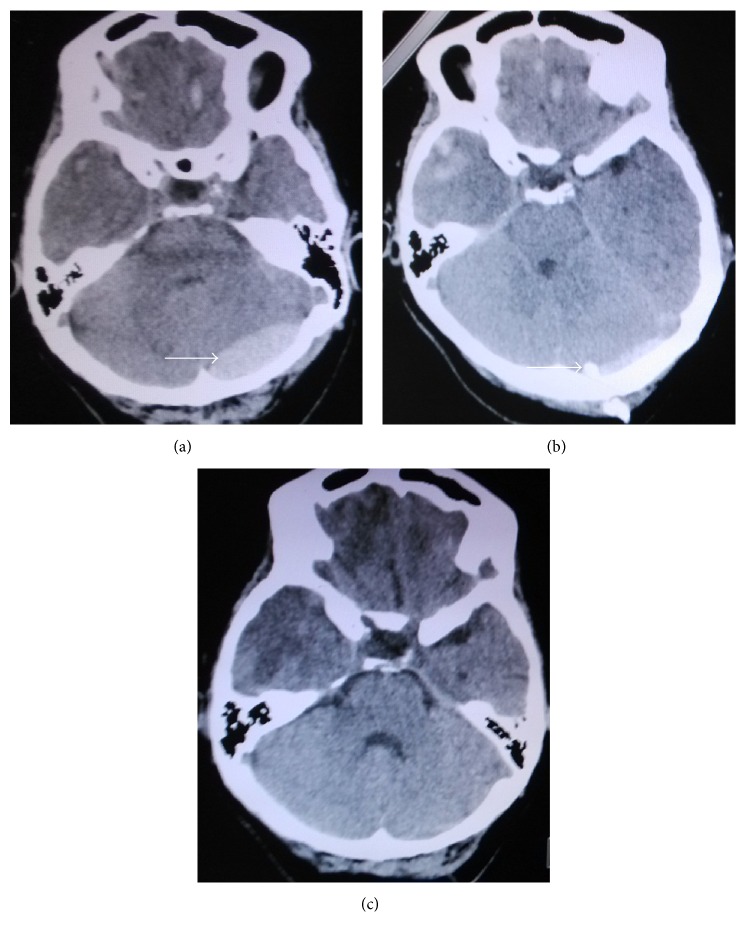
(a)–(c) Head CT images showing acute epidural hematoma at the left occipital region prior to and after surgery. (a) Head CT images prior to surgery; bleeding volume was approximately 13 mL. (b) Head CT images 3 days after surgery; hematoma volume was obviously reduced. Arrows indicate the site of hematoma and drainage tube. (c) Head CT images 5 days after surgery; hematoma was almost cleared, and the drainage tube was pulled off.
